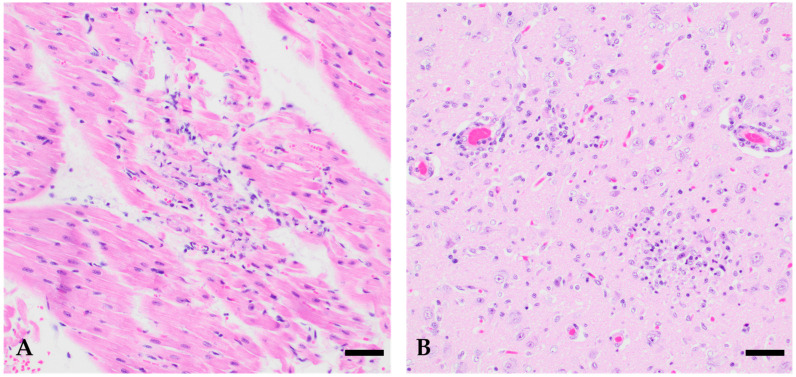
Histomorphological alterations caused by USUV in the heart and brain of two seals, (A) heart of case 4, with focal mild, acute, necrotizing, non-suppurative myocarditis, HE, (B) cerebrum of case 4 with signs of acute, non-suppurative, necrotizing encephalitis composed of multifocal mild perivascular lymphohistiocytic cuffing and glial nodules with central necrosis, HE, bar 20 µm.
